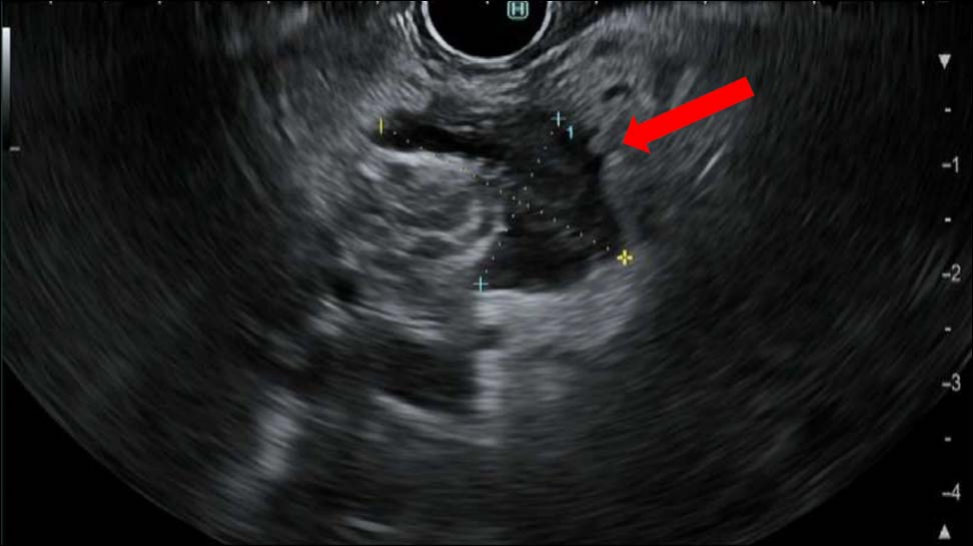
Endoscopic ultrasound showing an irregular fluid collection measuring 25 × 17 mm adjacent to the celiac axis.
